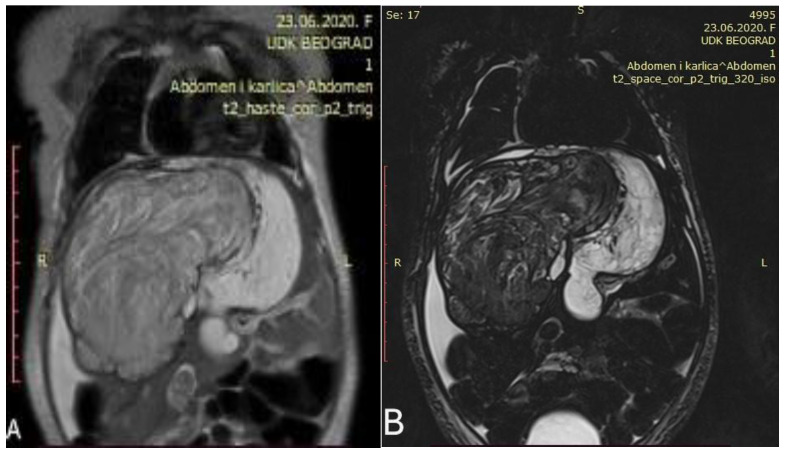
Initial abdominal MRI showed a voluminous heterogeneous tumor occupying the right liver ((A,B) Different coronal slices of the same MRI).
